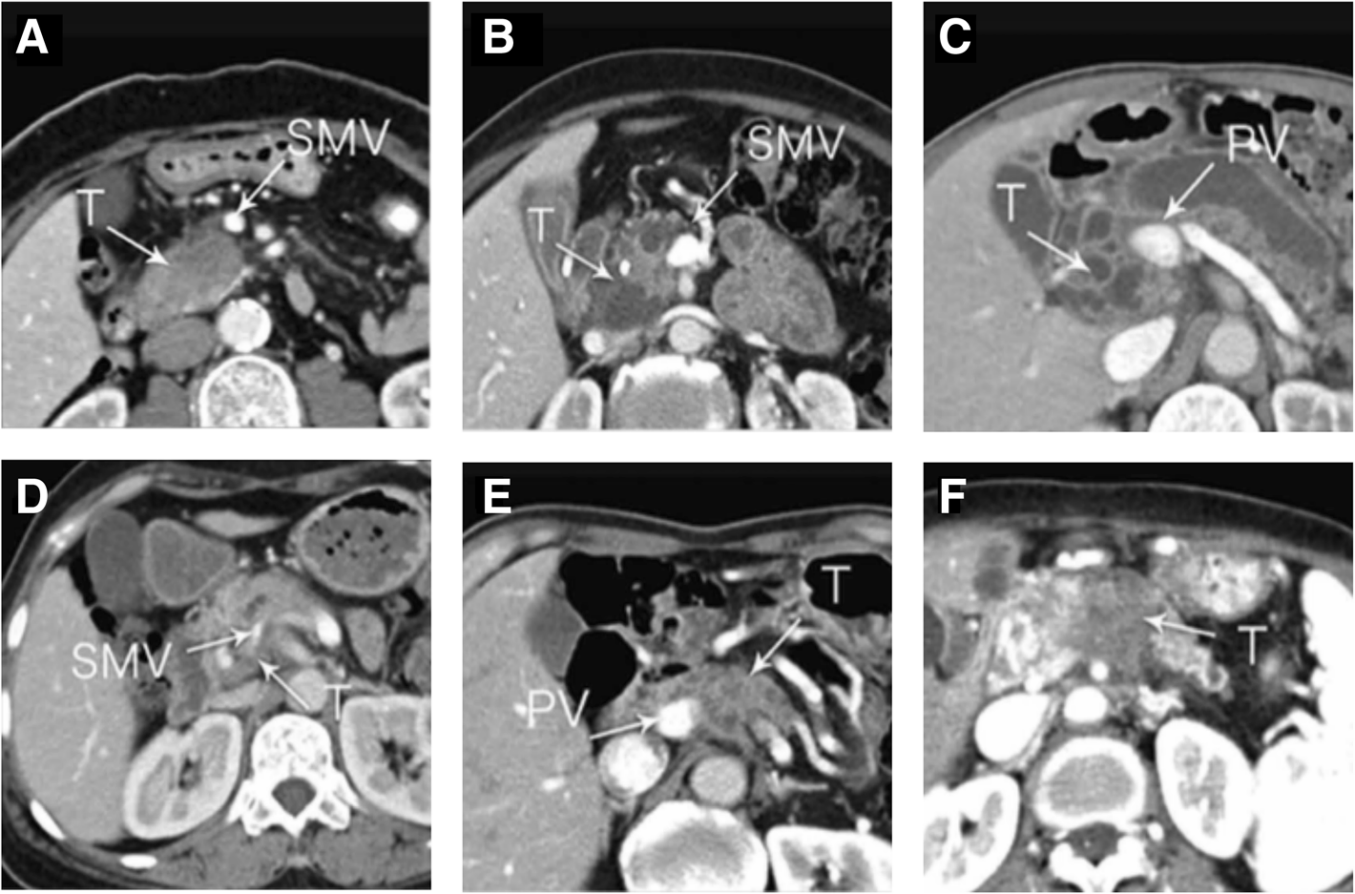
Venous contour abnormalities on CT. a, absent; b, tear drop; c, mild deformity; d, stenosis > 50%; e, tethering; f, obstruction
